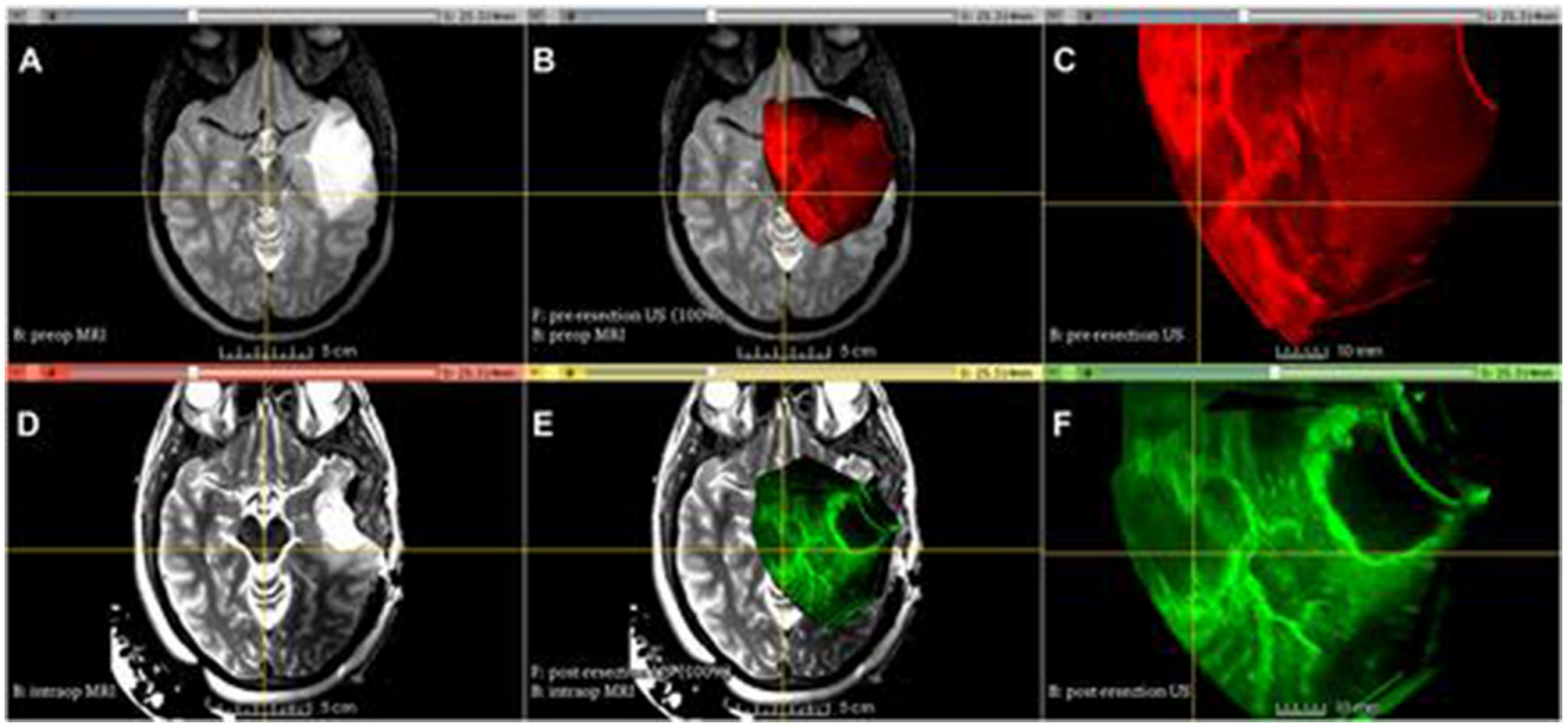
Advanced Multimodality Image Guided Operating (AMIGO) Suite at Brigham and Women's Hospital utilized tracked 3D US imaging and MRI during glioma resection. The following images show different images acquired during the procedure: (A) T2-fluid-attenuated inversion recovery (FLAIR) MRI before the operation, (B) 3D ultrasound before the durotomy, (C) 3D ultrasound before the durotomy, (D) 3D ultrasound before the durotomy, (E) 3D ultrasound during the operation, (F) 3D ultrasound after the initial tumor removal (29).
